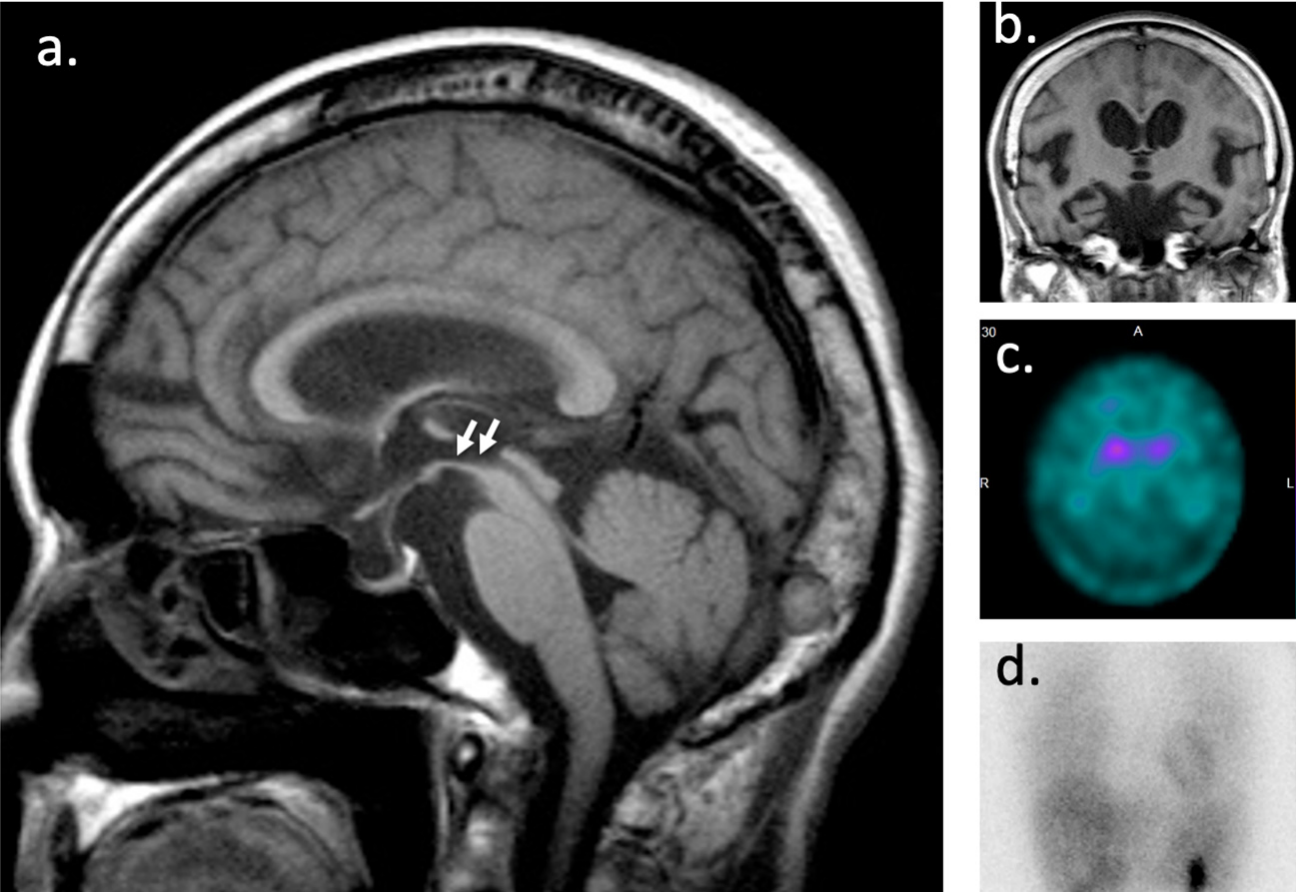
Progressive supranuclear palsy with disproportionately enlarged subarachnoid space hydrocephalus (DESH). (A) Sagittal section of brain magnetic resonance imaging (MRI) in a progressive supranuclear palsy (PSP) patient showing midbrain atrophy with preservation of the pons (below white arrows) known as the “hummingbird” sign. (B) Coronal section of brain MRI showing “DESH” sign. Dopamine transporter imaging with [^123^I] N-ω-fluoropropyl-2β-carboxymethoxy-3β-(4-iodophenyl) nortropane (FP-CIT) single-photon emission computed tomography (SPECT) images (C) and ^123^I-meta iodobenzylguanidine (MIBG) cardiac scintigraphy (D). PSP patients have a significantly lower uptake of FP-CIT in the caudate nucleus and putamen than iNPH patients and normal individuals. In PD and LBD, there is nearly no MIBG uptake in the myocardium, while normal MIBG uptake in the myocardium is shown in PSP. iNPH – idiopathic normal pressure hydrocephalus; PD – Parkinson’s disease; LBD – Lewy body dementia.
